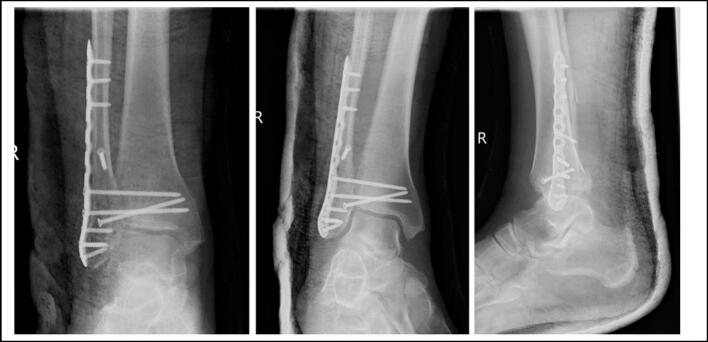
Initial post operative AP, mortice and lateral radiographs at 2 weeks post op.
